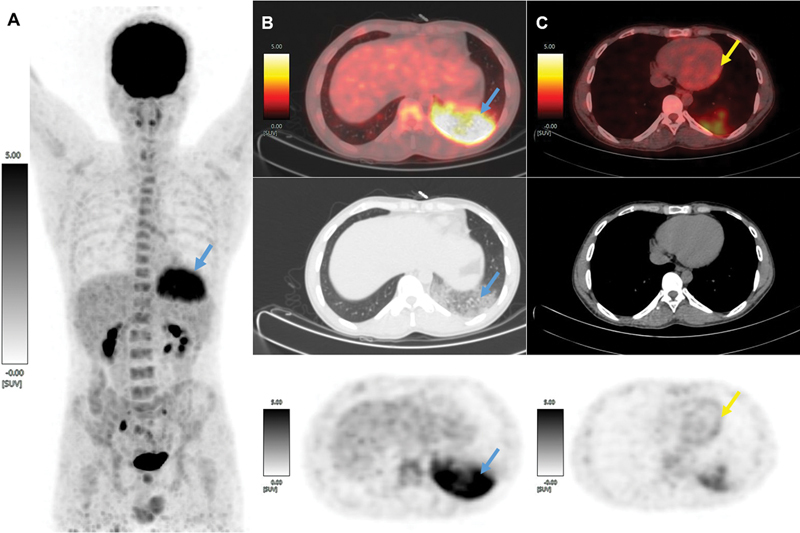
Maximum intensity projection of F-18 2-fluoro 2-deoxyglucose positron emission tomography with noncontrast computed tomography ([
